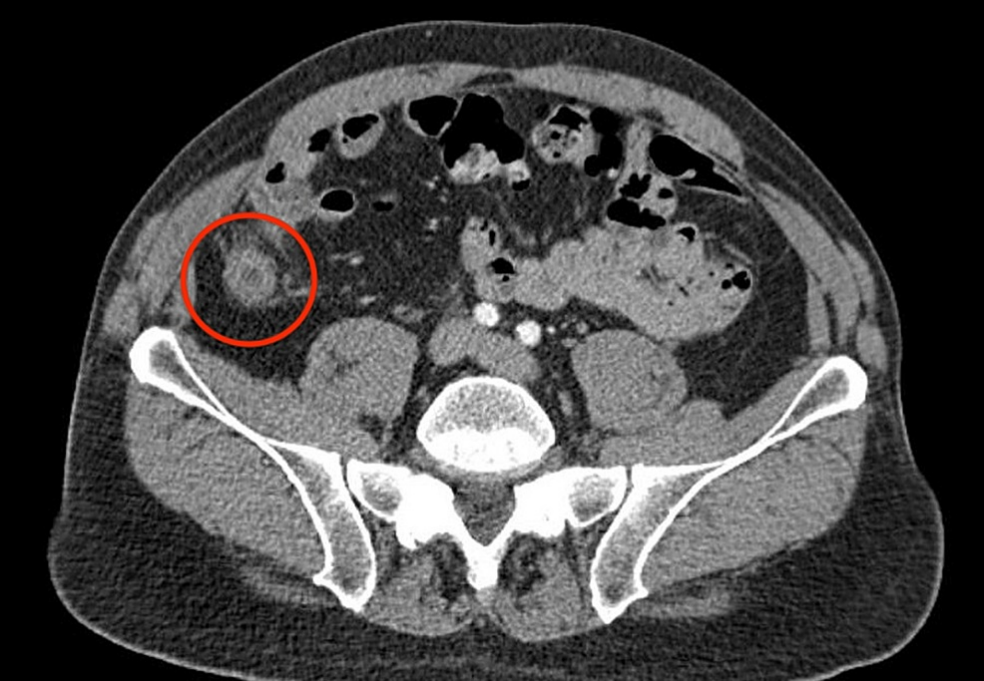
CT scan image (transverse view) showing appendiceal wall thickening.
